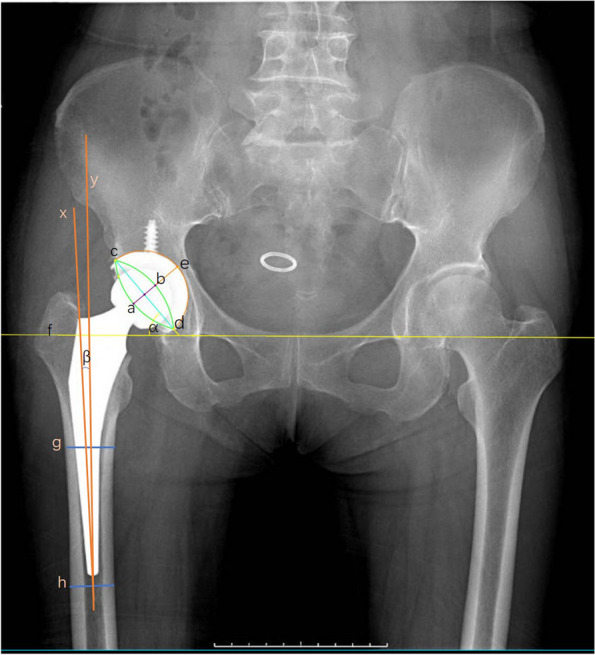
Widmer’s method (Anteversion = 48.05 × ab / ae – 0.3) measures the anteversion of the acetabulum on X-rays. “α” and “β” represent the abduction angle of the acetabulum and the eversion angle of the femoral stem, respectively. “X” is the longitudinal axis of the femoral stem, and “Y” is the longitudinal axis of the proximal femoral cavity
